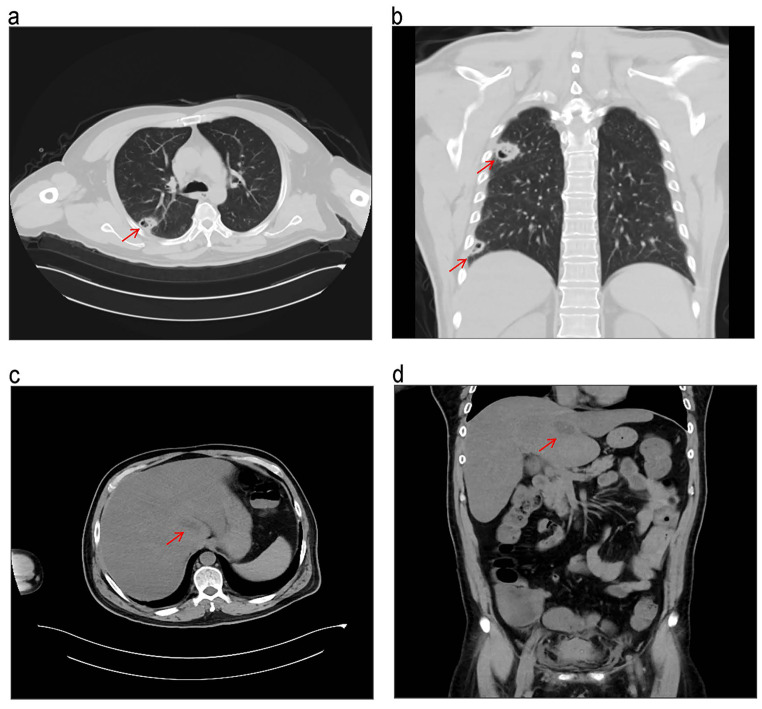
CT scans taken one day after admission demonstrating lung and liver findings. (a) and (b) Lung CT scans at admission show multiple nodules and patchy shadows across all lobes of both lungs. The most significant nodule, located in the lower lobe of the right lung, has a cross-section measuring about 3 cm × 2.2 cm, as indicated by the red arrow. (c) and (d) Hepatic CT scans reveal an increased volume of the caudate lobe and a low-density area(3.0 cm × 1.3 cm) in the caudate liver lobe, highlighted by the red arrow
